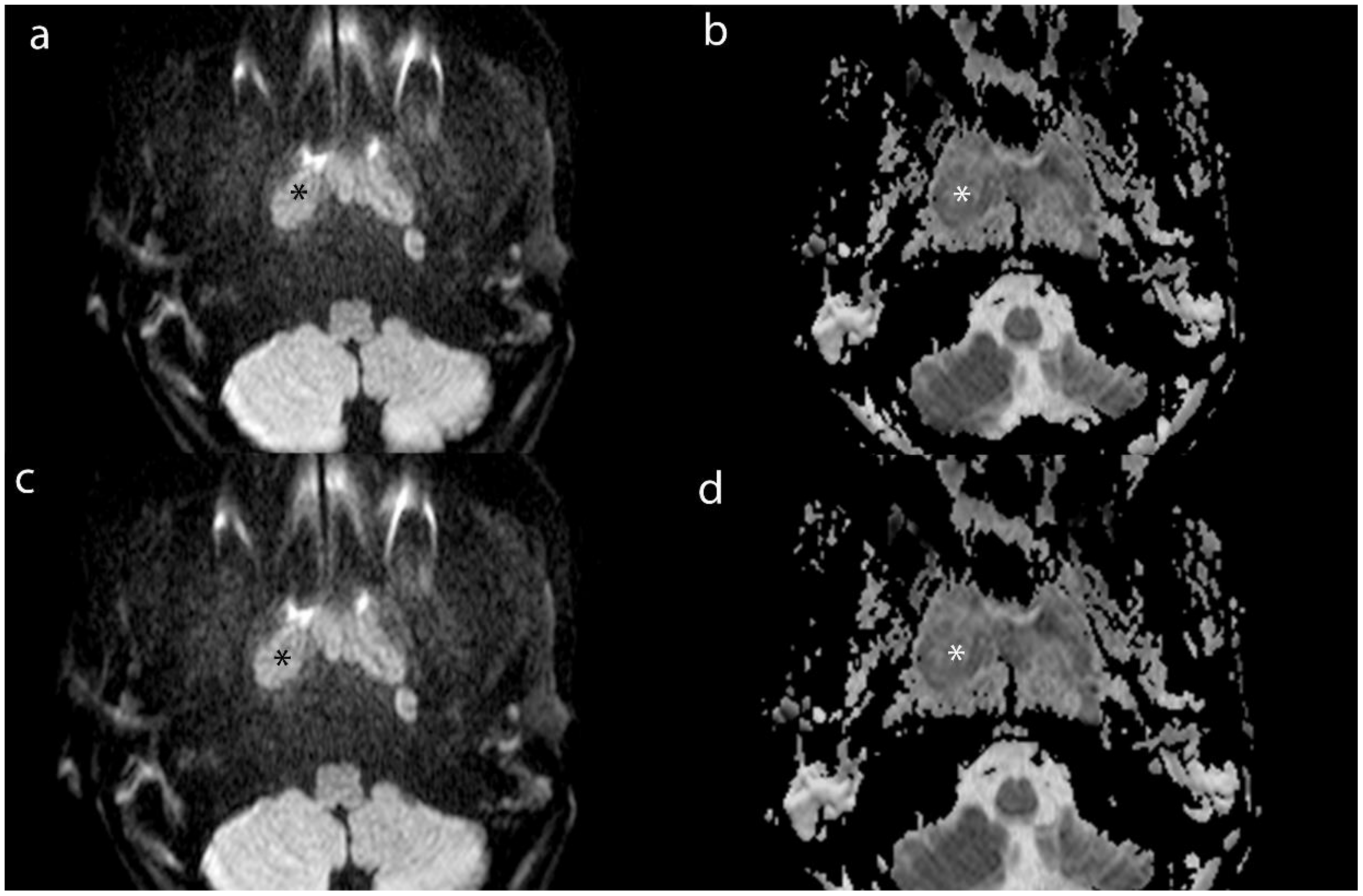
Axial MR images of lesion (*) and MLN (*) from a 16-year-old male patient with NPC. (a) and (b) show the DWI and ADC images of lesion. (c) and (d) show the DWI and ADC images of MLN.
